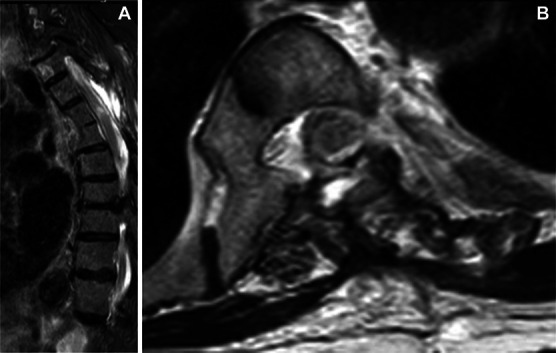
A, Sagittal and B, axial postoperative T2-weighted MRI showing complete tumor removal.
